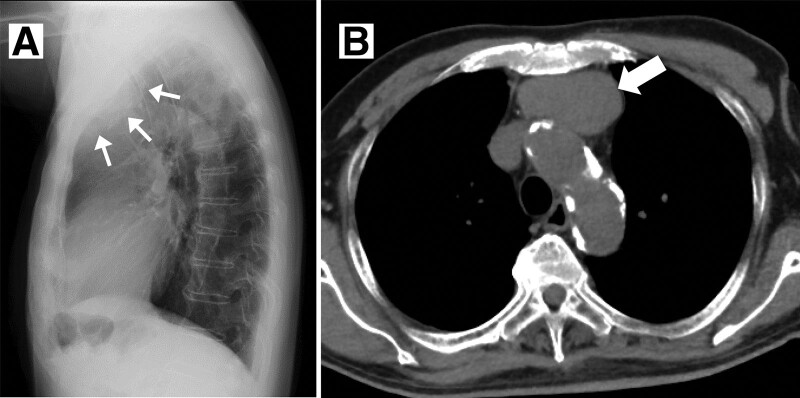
(A) Lateral view of chest X-ray. A mass lesion (arrow) from the ascending aorta to the aortic arch. (B) Computed tomography of the chest revealing a well-defined mass lesion (arrow) of 60 mm in the anterior mediastinum. The mass lesion tended to increase; thus, a noninvasive thymoma was suspected.
